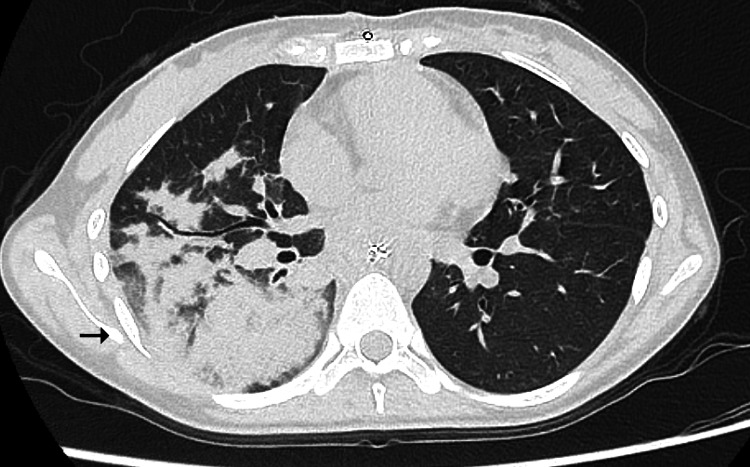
Computed tomography (CT) scan obtained after reintubation revealed massive consolidation predominantly in the right lower lobe (arrow)
